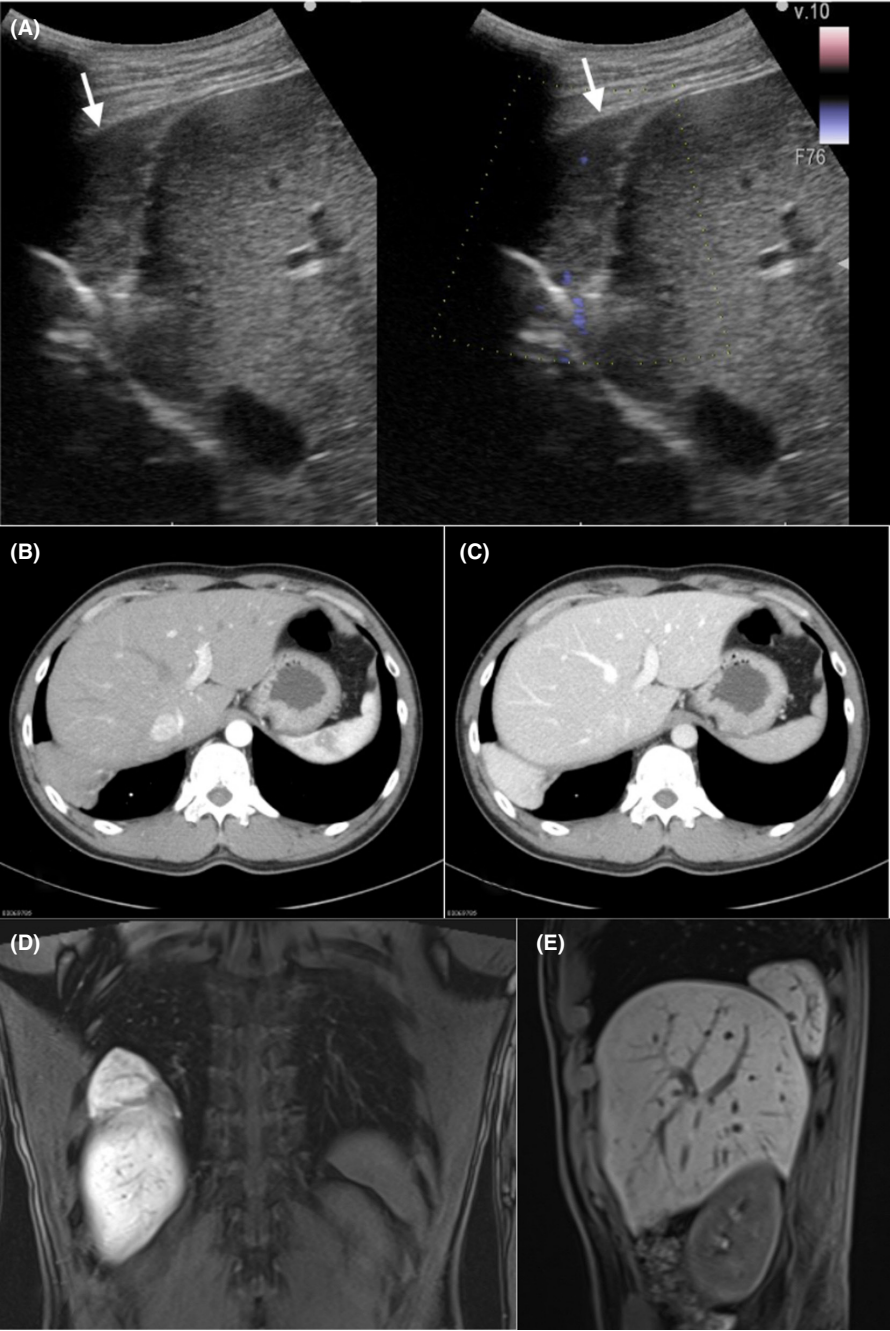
Abdominal ultrasonography (A). The mass is seen in the right costal region (arrow). The mass seems to be separated from the liver, but the echogenic level is similar to that of the liver. Vascular structures are seen inside the mass. Contrast‐enhanced computed tomography. (B): arterial phase. (C): late portal phase. The mass is seen in the lower right thorax. Blood from the mass flows into the portal vein, but no afferent arteries are visible. Its pattern of contrast uptake is similar to that of the liver. Contrast‐enhanced magnetic resonance imaging in the hepatocellular phase. (D): coronal view. (E): sagittal view. The mass is seen in the lower right thorax. Its pattern of contrast enhancement is similar to that of the liver
